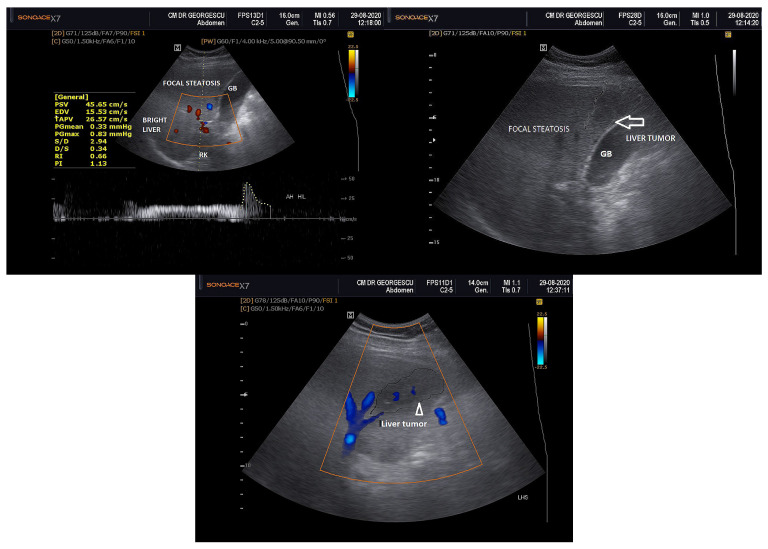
Ultrasound examination of the liver. (Left): Triplex exam demonstrates the bright right liver lobe, large focal steatosis, the patency of the portal vein and a mild elevation of the RI of the hilar hepatic artery. (Right): 2D exam, arrow pointing at the left lobe’s tumor surrounded by the focal steatosis. (Middle): Duplex exam, tip of the arrow pointing at left lobe’s tumor surrounded by the focal steatosis. (GB = gallbladder, RK = right kidney).
